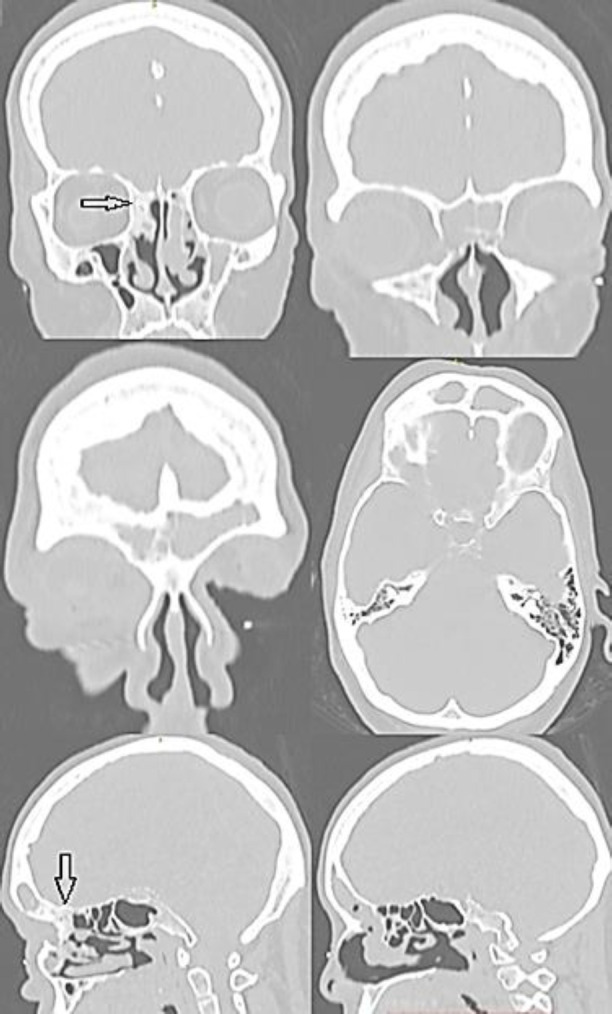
Paranasal sinuses CT scan showing a 25×15×10 mm (height-length-width) bony mass (black arrow) arising from the right frontoethmoidal area which causes total opacification of the right frontal sinus and right frontal sinus anterior wall defect with orbital cellulitis. Left maxillary sinus total opacification
